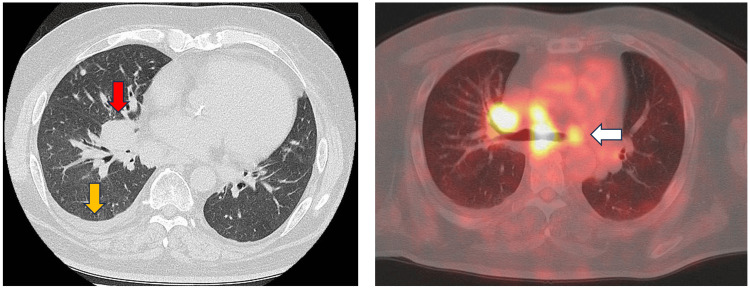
Imaging findings at the initial visit Left image: Computed tomography (CT) scan before first-line chemotherapy showing the right hilar tumor of approximately 4 cm in size (red arrow) and right pleural effusion (yellow arrow). Right image: PET-CT also revealed positive lymph node metastasis in the contralateral mediastinum (white arrow). PET: Positron emission tomography.
